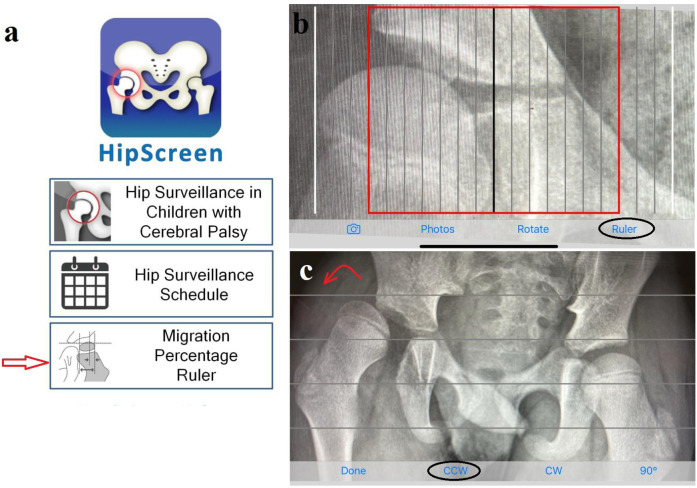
a) Home screen of the HipScreen (HS) app with a red arrow pointing to the ruler function. b) Ruler with the red line indicating 30% migration percentage. c) Rotate function to correct pelvic obliquity; in this radiograph, counter clockwise (CCW) is needed (red curved arrow). Permission obtained from original authors.^12^
